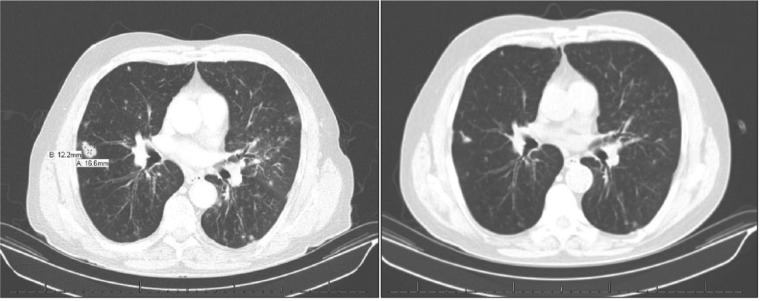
Left: CT chest on presentation. Note the diffuse poorly defined nodules and ground glass opacities. Right: CT chest 9 months after cessation of cosmetic talc use, demonstrating resolution of ground glass opacities and smaller, less dense nodules.
